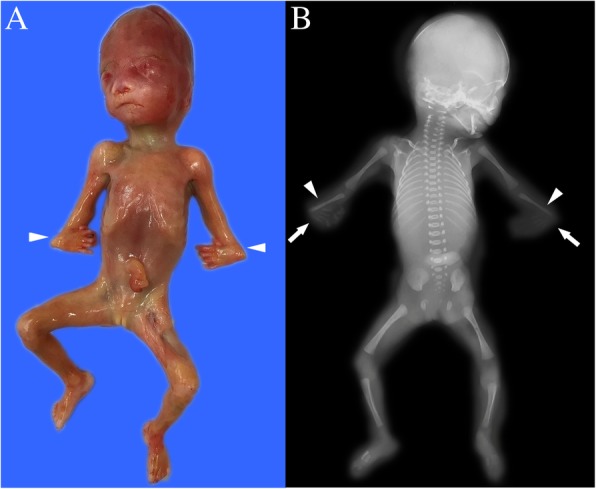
Case 2: The fetus with trisomy 18 and the absence of the radius. a Gross anatomy of the aborted fetus, showing the bilateral wrist flexion (arrowheads). b X-ray image of the aborted fetus, showing the bilateral bone defects (arrowheads) and bilateral wrist flexion (arrows)
